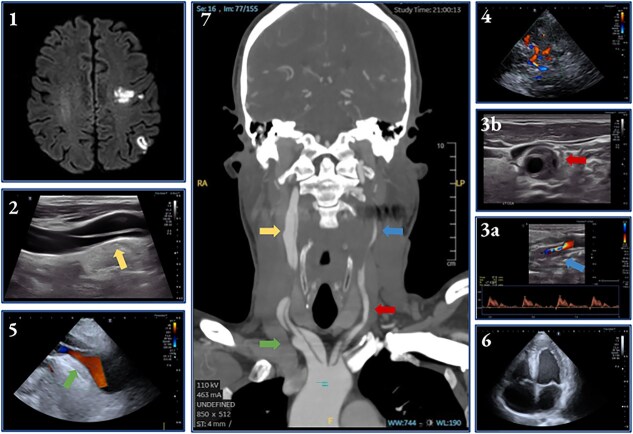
A 54-year-old man with a history of hereditary spherocytosis presented with mild aphasia and headache that began 24 hours earlier. Brain MRI showed subacute ischemic lesions in the left parietal region on diffusion-weighted imaging (1). Brain POCUS, performed in the stroke unit, revealed dissections in both common and internal carotid arteries, including a mobile dissecting membrane and a double lumen on the right side (2), along with a vessel wall hematoma (3a), and distal occlusion (3b). Transcranial Doppler showed reversed blood flow in the left A1 segment of the anterior cerebral artery indicating collateral circulation through the anterior communicating artery (4). Cardiac POCUS identified extension of the dissection from the brachiocephalic trunk (5) and features consistent with restrictive cardiomiopathy (6). CT angiography confirmed supraortic and cervical vessel findings (7).
